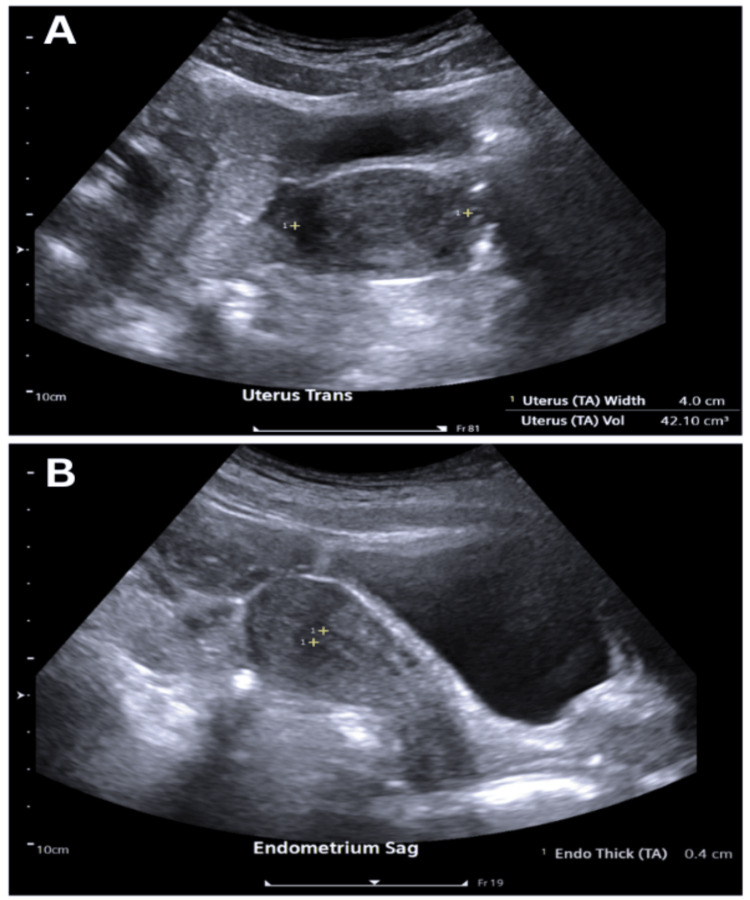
Pelvic ultrasound demonstrating normal uterine anatomy and endometrial thickness (A) Transverse view of the uterus showing normal contour and homogeneous myometrium. (B) Sagittal endometrial view showing thickness appropriate for menstrual phase without a focal intracavitary lesion. (Ultrasound performed on day 6 of the menstrual cycle; endometrial thickness measured as 0.4 cm (4 mm) as shown in Figure 1B.)
